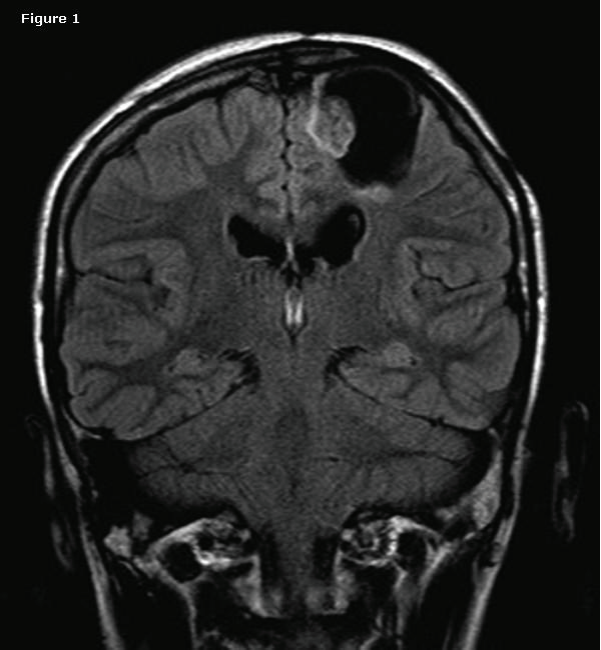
Coronal FLAIR image shows approximately 3 × 4 cm in diameters cystic mass in the left frontal lobe. Cystic mass has a nodulary portion with different signal
